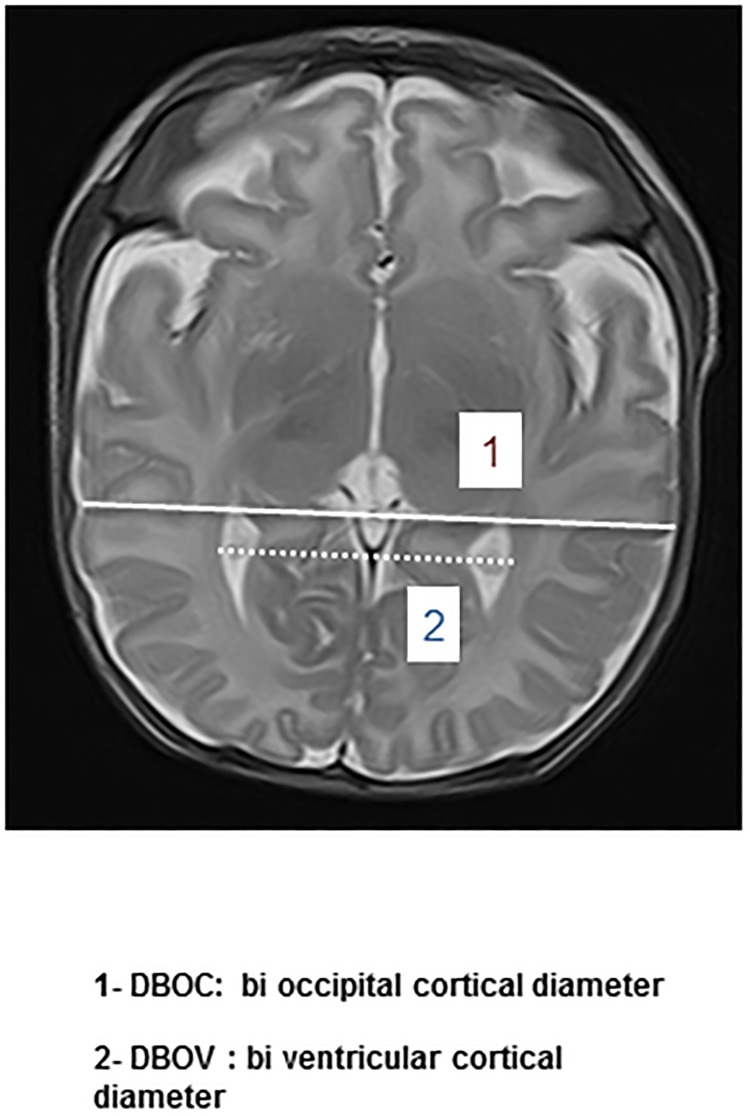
MRI linear indices on the axial image in T2-weighted including mesencephalon and occipital horn of lateral ventricle, below the thalamic nuclei. DBOv: bi occipital ventricular diameter / DBOc: bi occipital cortical diameter.
